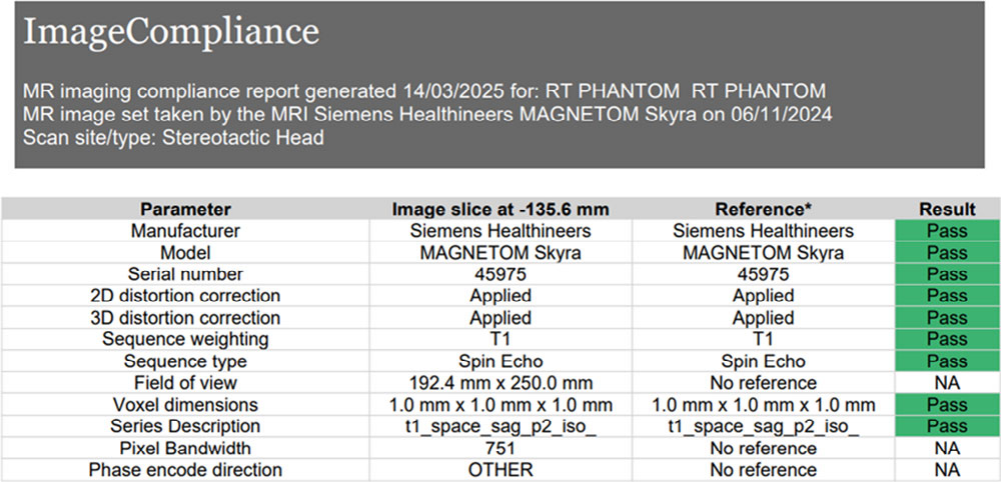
Sample MRI QA report generated by ImageCompliance V2.0.
